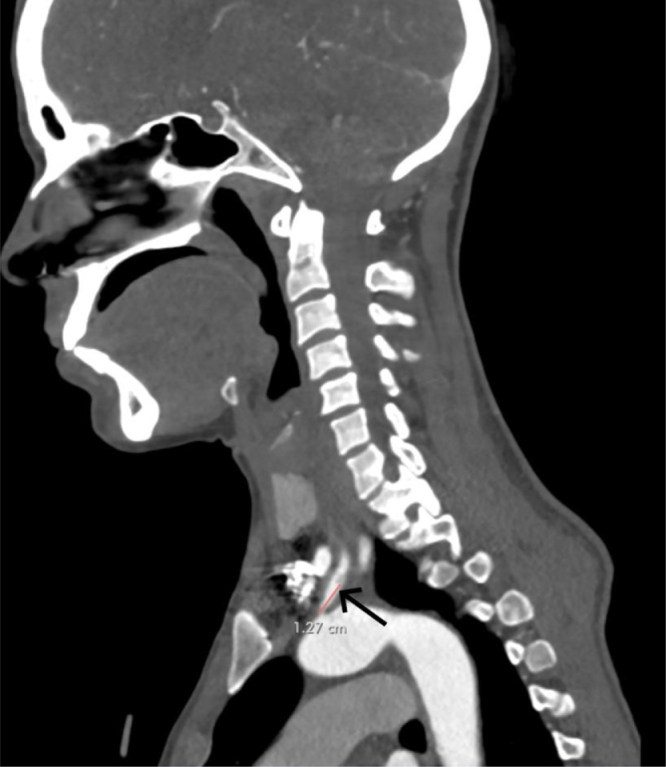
Sagittal computed tomography angiography image of the neck demonstrating luminal irregularity of the extracranial left vertebral artery with a linear intimal flap (arrow) approximately 1.27 cm from its origin, consistent with vertebral artery dissection.
